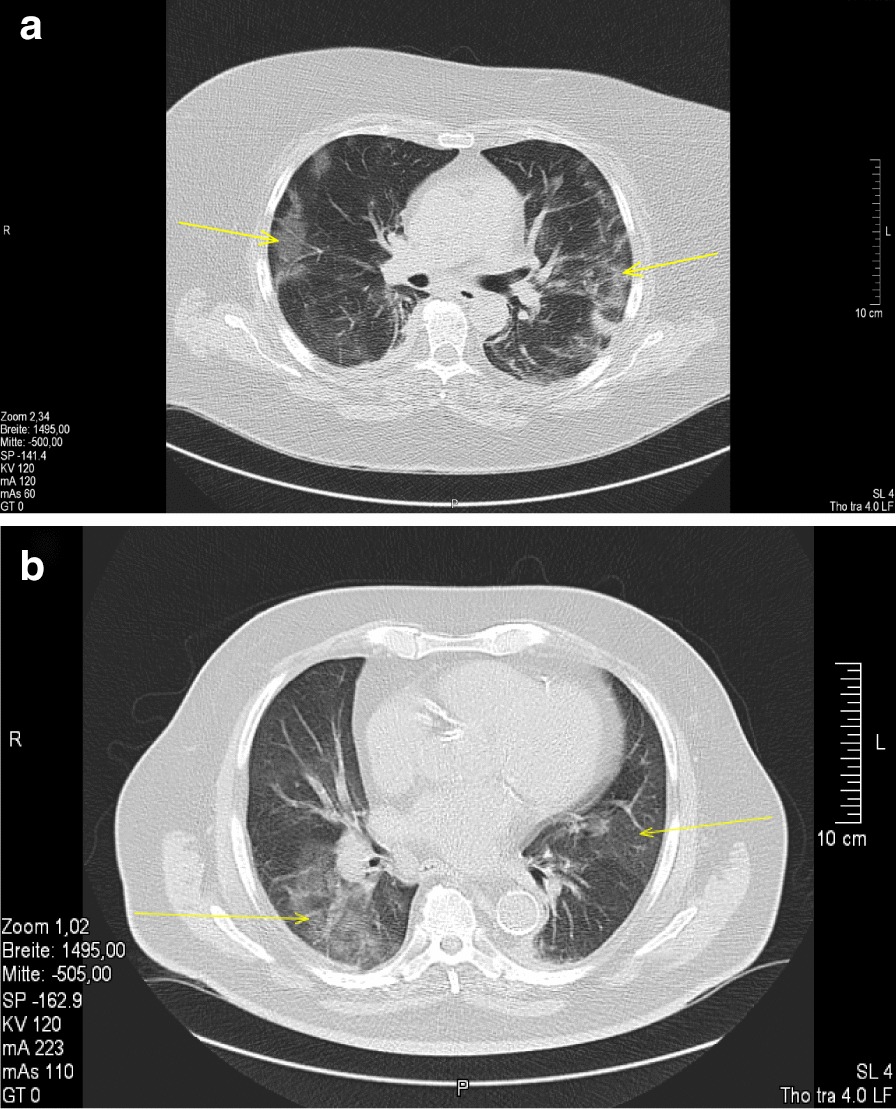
Thoracic computed tomography showing bilateral ground-glass opacities (arrows) of patient 1 (a) and patient 2 (b)
